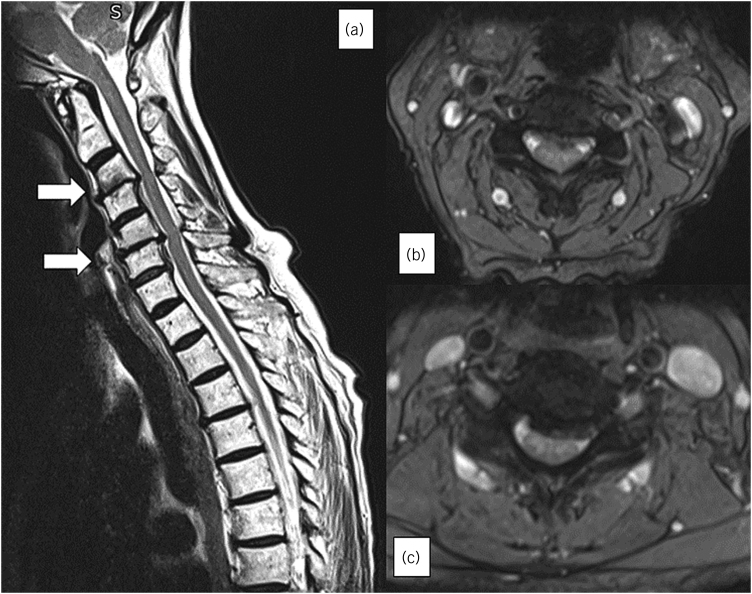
(a) Canal stenosis at levels C3/4 and C5/6. (b, c) Axial MRI T2 cuts of the levels respectively. MRI = magnetic resonance imaging
